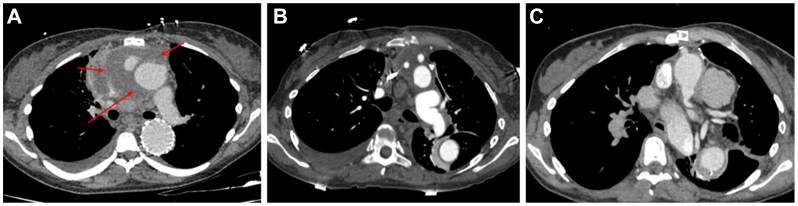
Computed tomography evolution of prosthetic aortic graft infection and postoperative reconstruction. Contrast-enhanced computed tomography angiography (CTA), axial views. (A) At admission, extensive mediastinal infiltration and circumferential periprosthetic fluid collection surrounding the ascending aortic graft, consistent with purulent mediastinitis (*red arrows*). (B) CTA 22 days after complete explantation of the infected Thoraflex and cTAG prostheses and in situ reconstruction with cryopreserved aortic allografts, showing patent reconstruction and resolution of mediastinal collections. (C) Four-month follow-up CTA demonstrating stable allograft reconstruction and absence of residual infection.
